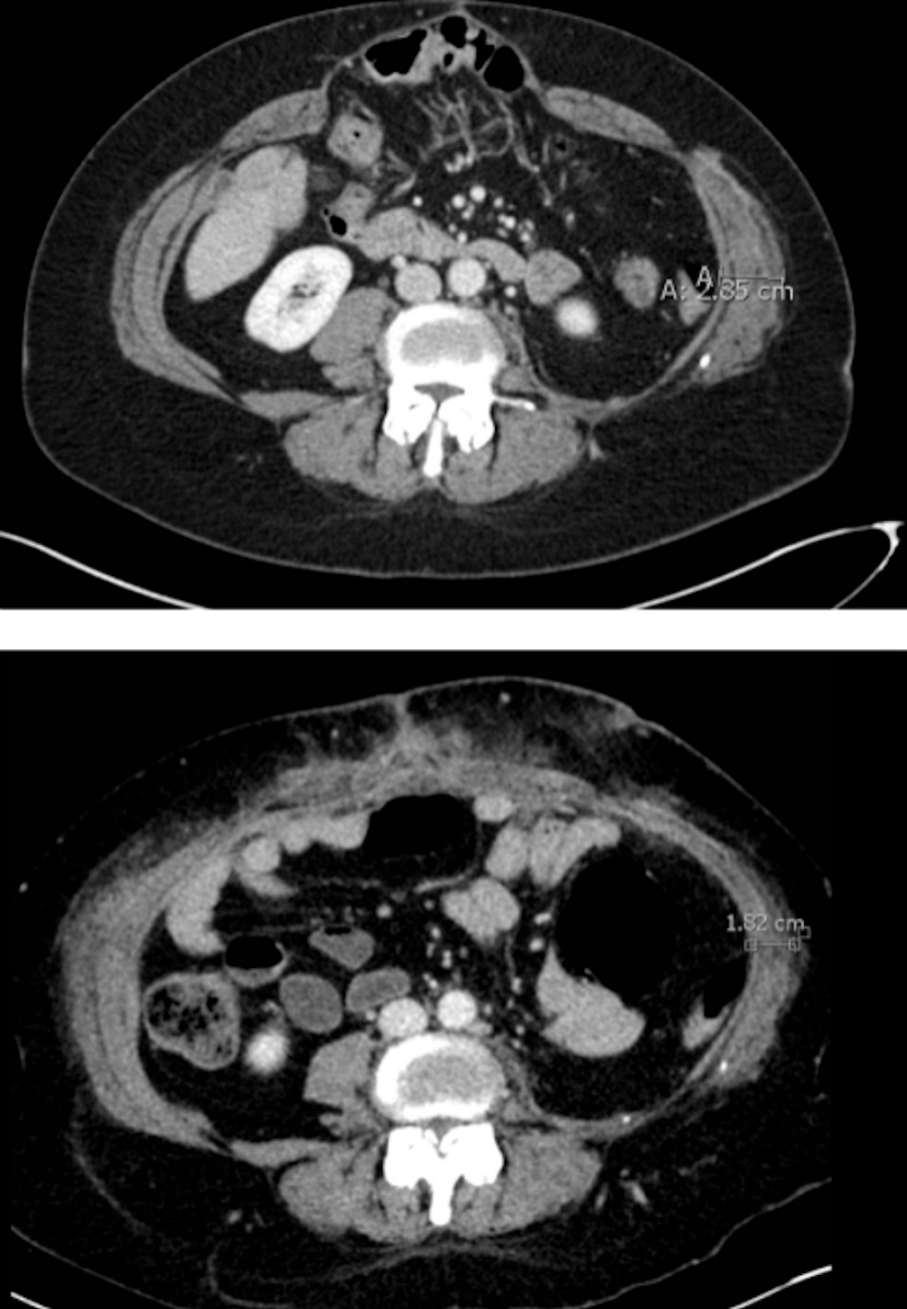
Pre- and Post-Botox Injection CT Scans These CT scans of a cross-section of the abdomen show how the Botox enabled us to pull her abdominal muscles back into position. In the top image, the hernia is visible before surgical intervention. Measurement shows that the right abdominal muscle has retracted and consequently has thickened to a width of 2.85 cm. In the lower image, the muscle has been stretched thinner, so the new measured width is 1.82 cm, and the muscle was pulled back to the center of her body.
